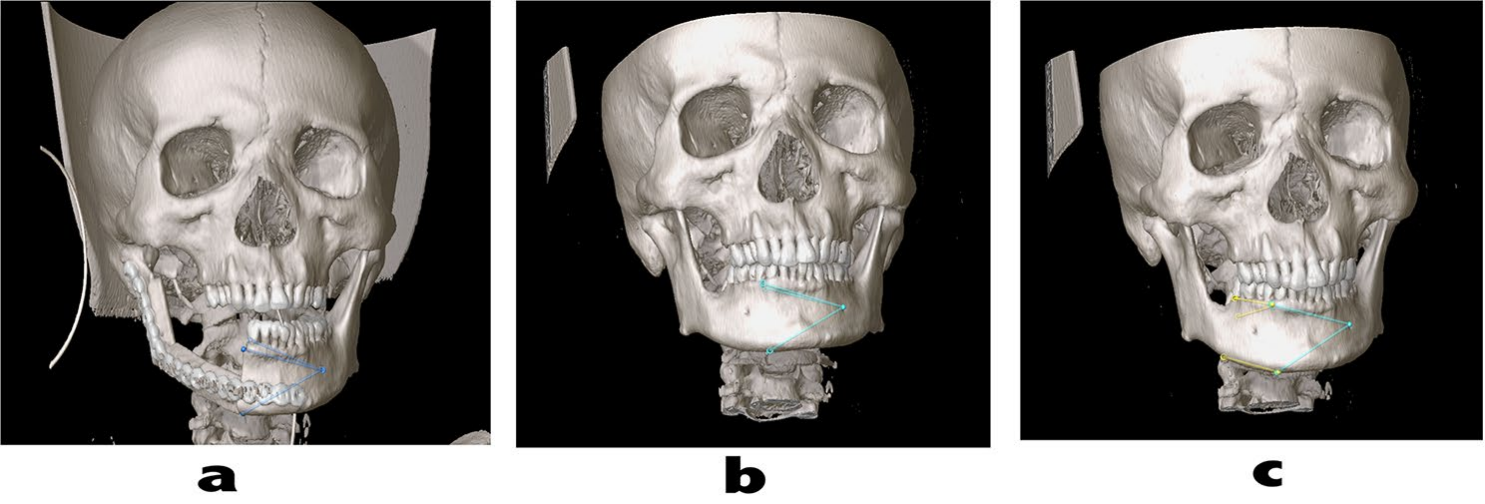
The pathological sample was located on the computerized reconstruction image. a The osteotomy location is marked on the postoperative CT image, and the position relationship between the points and the chin foramen is recorded. b The position relationship between each point and the chin foramen was used to correct the position deviation of the mandible, and the osteotomy position was located on preoperative CT. c The yellow path points to the material location
